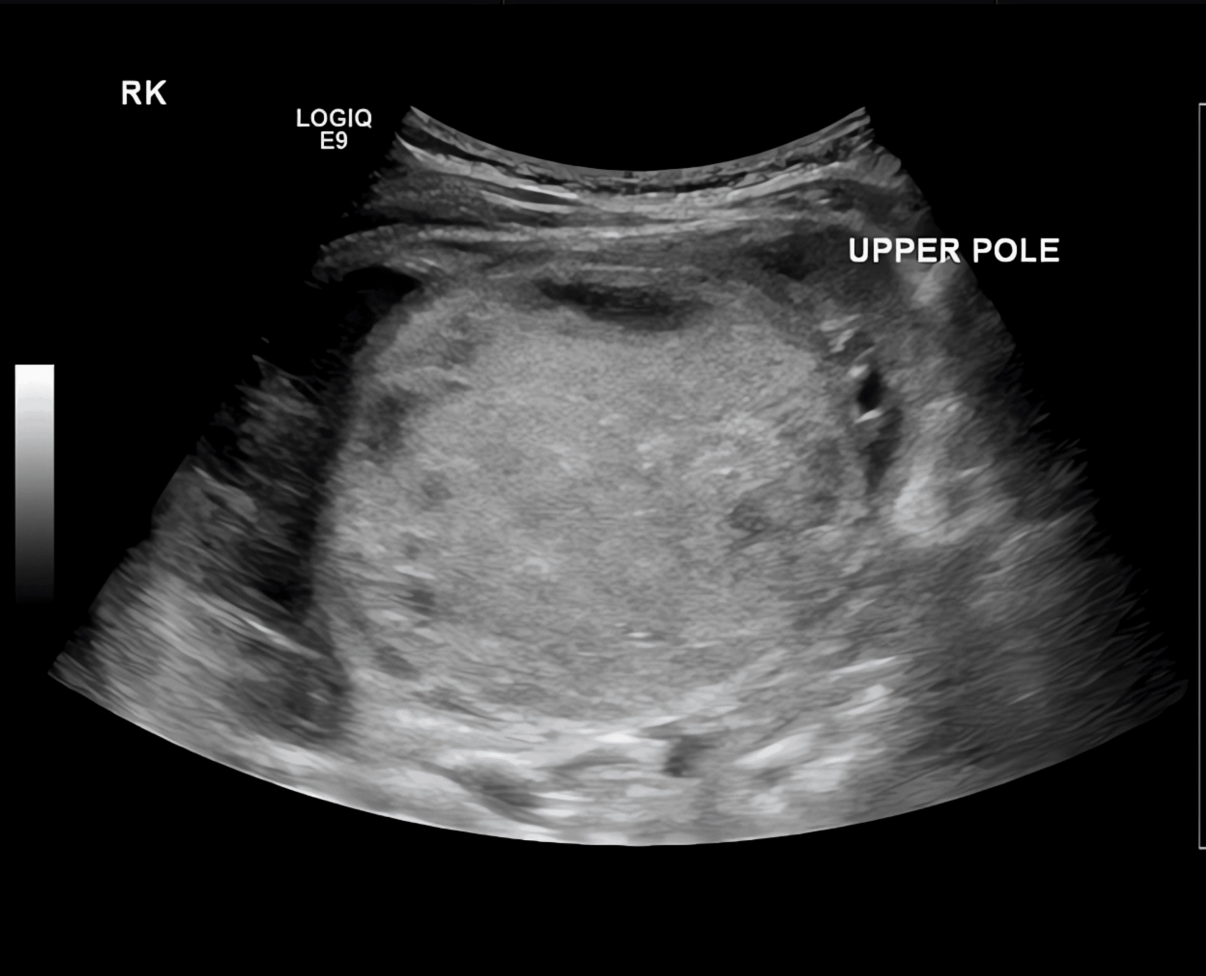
Ultrasound image of the right kidney showing a predominantly echogenic renal mass with small cystic/necrotic areas and mild hydronephrosis
